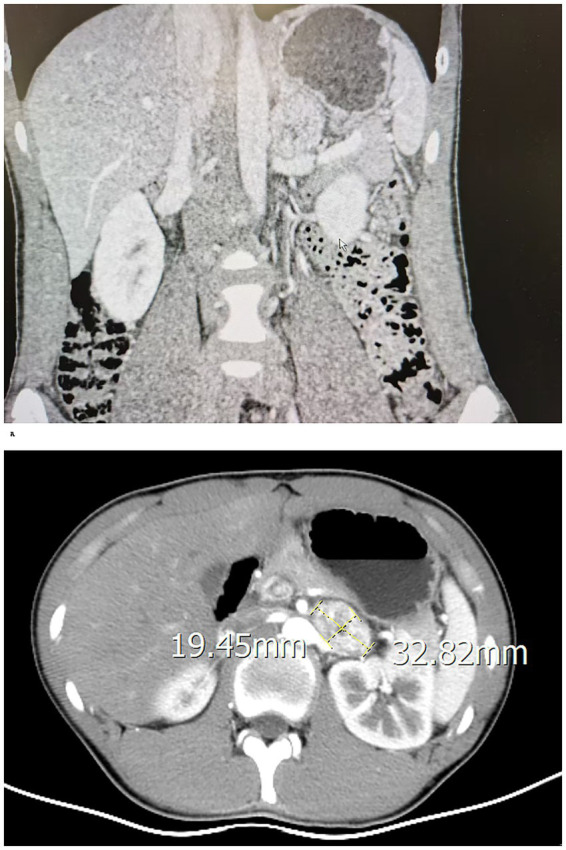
Preoperative abdominal CT showing a 3.3 × 2.0 cm left adrenal pheochromocytoma.
